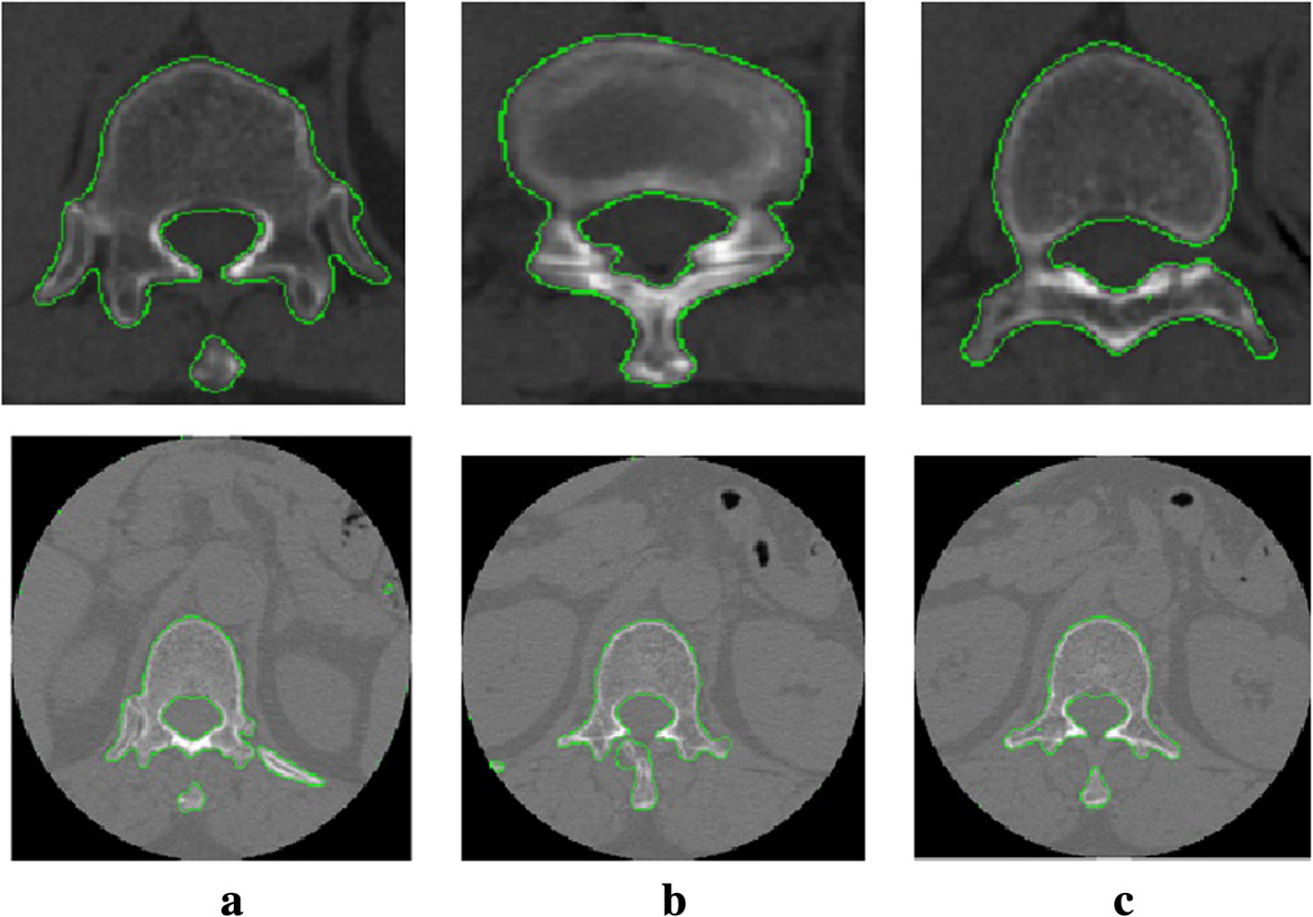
Neighboring slices are similar in 2D CT image data set. (a) image slice 31; (b) image slice 32;(c) image slice 33; (d) image slice 34.
